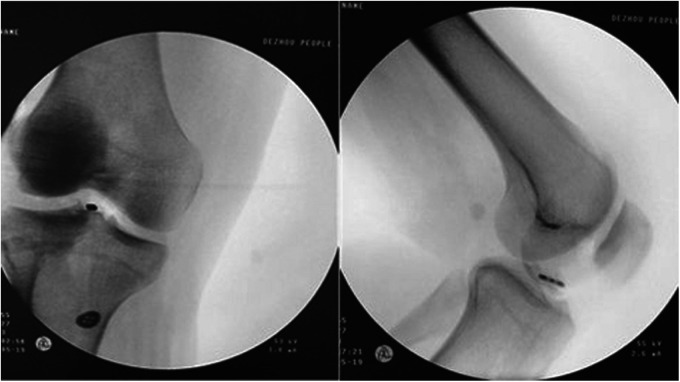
Postoperative anterior-posterior and lateral view radiographs. The ideal position (A).
